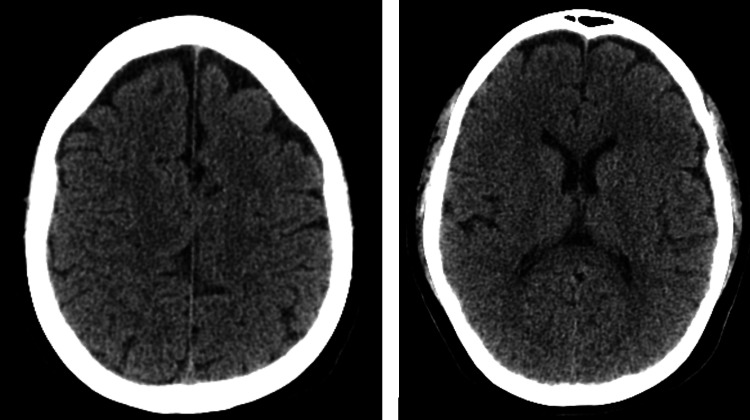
Follow-up cranial CT showing partial resolution of the parasagittal parietal and frontoparietal hygromas.
